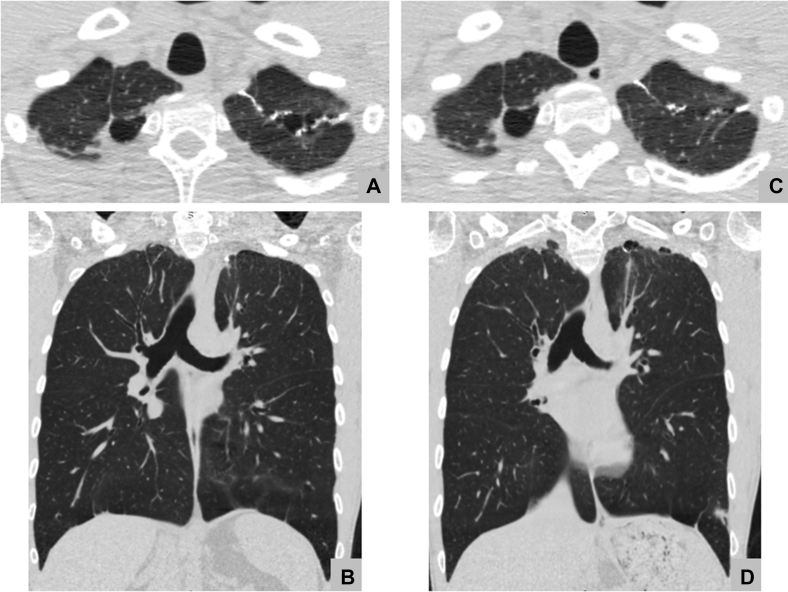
A-D, Chest high-resolution CT scan at 1-year follow-up in our center: (A) axial and (B) coronal reconstructions at follow-up showing stable apical pleural thickening and unchanged pleural and subpleural findings, and (C) axial and (D) coronal reconstructions obtained 1 y earlier, included for comparison.
